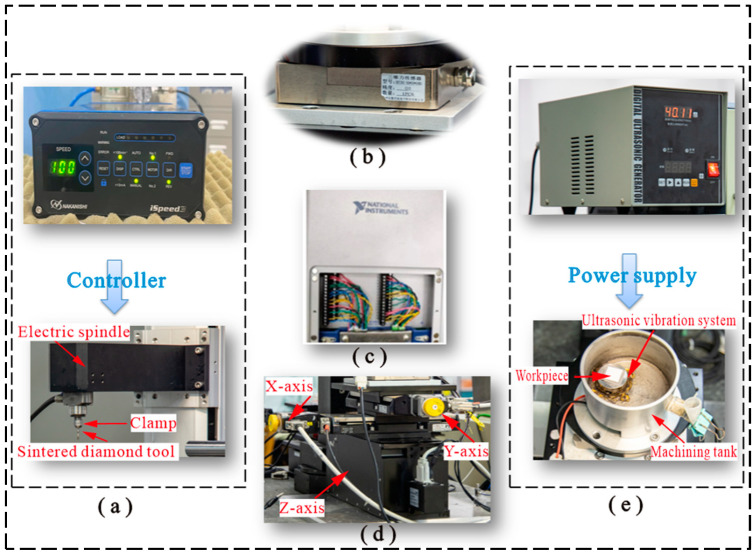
Design of key components: (a) NSK electric spindle system; (b) 3D pressure sensor; (c) NI DAQ card; (d) micro 3D motion platform; (e) ultrasonic vibration system workbench: machining tank and ultrasonic power supply.
